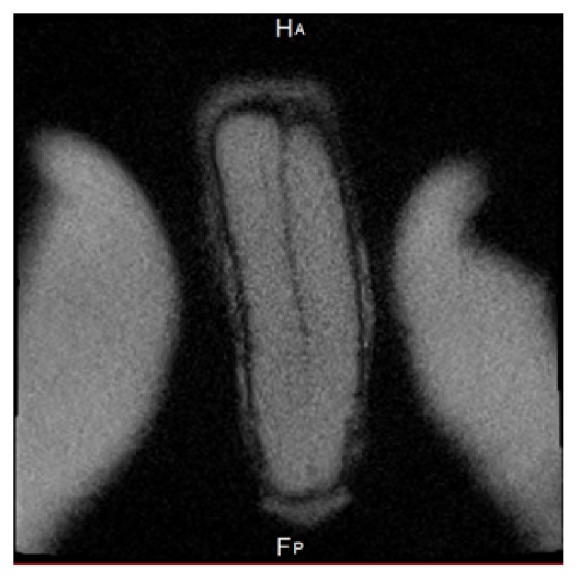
MRI of the penis showing the integrity of the cavernous bodies.
